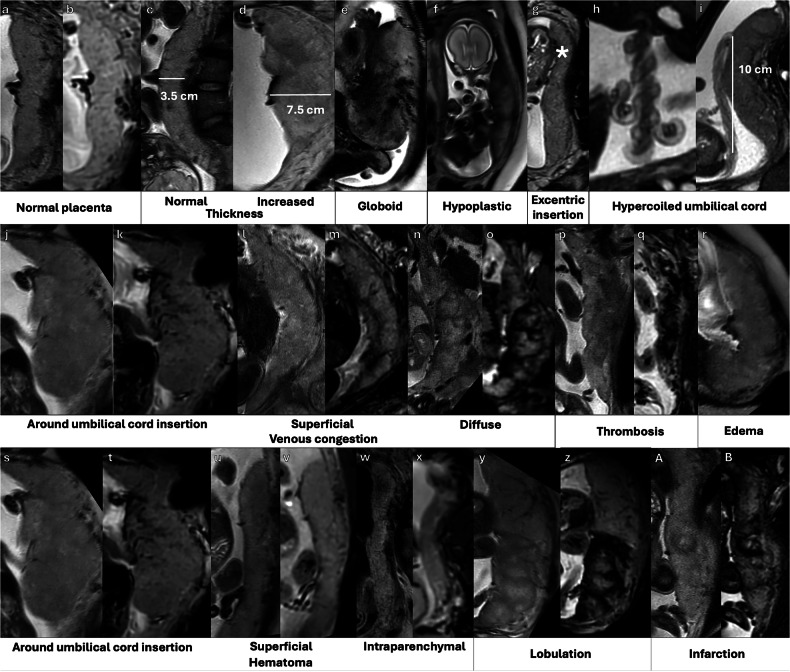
Exemplary placental anomalies depicted in T2-weighted and EPI/T2*-weighted imaging. a, b Normal placenta (21 + 2 GW). c, d Normal (c, 28 + 4 GW) and increased (d, 24 + 2 GW) placental thickness. e Globoid placenta shape (28 + 2 GW). f Hypoplastic placenta (24 + 5 GW). g Asterix indicating an eccentric insertion of the umbilical cord (26 + 4 GW). h, i Hypercoiled umbilical cord (25 + 4 and 20 + 1 GW). j–o Venous congestion of the placenta—around the insertion of the umbilical cord (j, k, 22 + 2 GW), in superficial placenta parts (l, m, 33 + 1 GW), and with diffuse involvement of the placenta (n, o, 25 + 2 GW). p, q Thrombosis of the placenta (24 + 0 GW). r Placental edema (22 + 2 GW). The presence of edema does not allow for assessment of lobulation. s–x Placental hematoma—around the insertion of the umbilical cord (s, t, 22 + 2 GW), in superficial placenta parts (u, v, 22 + 6 GW), and with diffuse involvement of the placenta (w, x, 22 + 0 GW). y, z Placental lobules (and diffuse venous congestion) in the lower placenta in twin pregnancy (24 + 3 GW). Edematous placenta of the second twin on top. A, B: Placental infarction (30 + 3 GW). GW, gestational weeks
